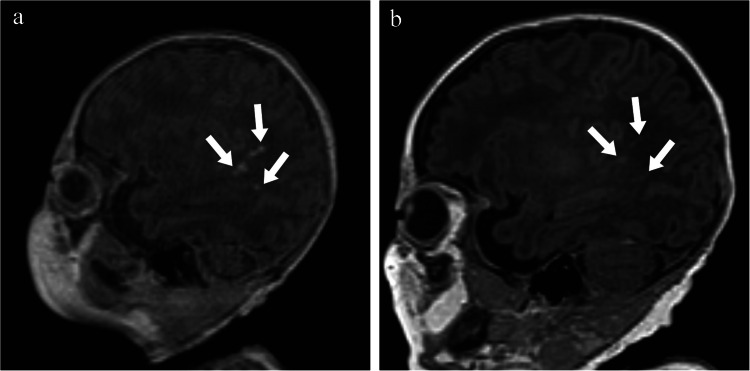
T1 parasagittal magnetic resonance images without contrast of a boy born at 35 + 6 weeks. a The image obtained on day 8 (37 + 0 weeks) shows multiple areas of hyperintensity indicating severe white matter injury (*arrows*). b The image obtained on day 45 (41 + 2 weeks) shows decreased intensity and size of all lesions (*arrows*)
